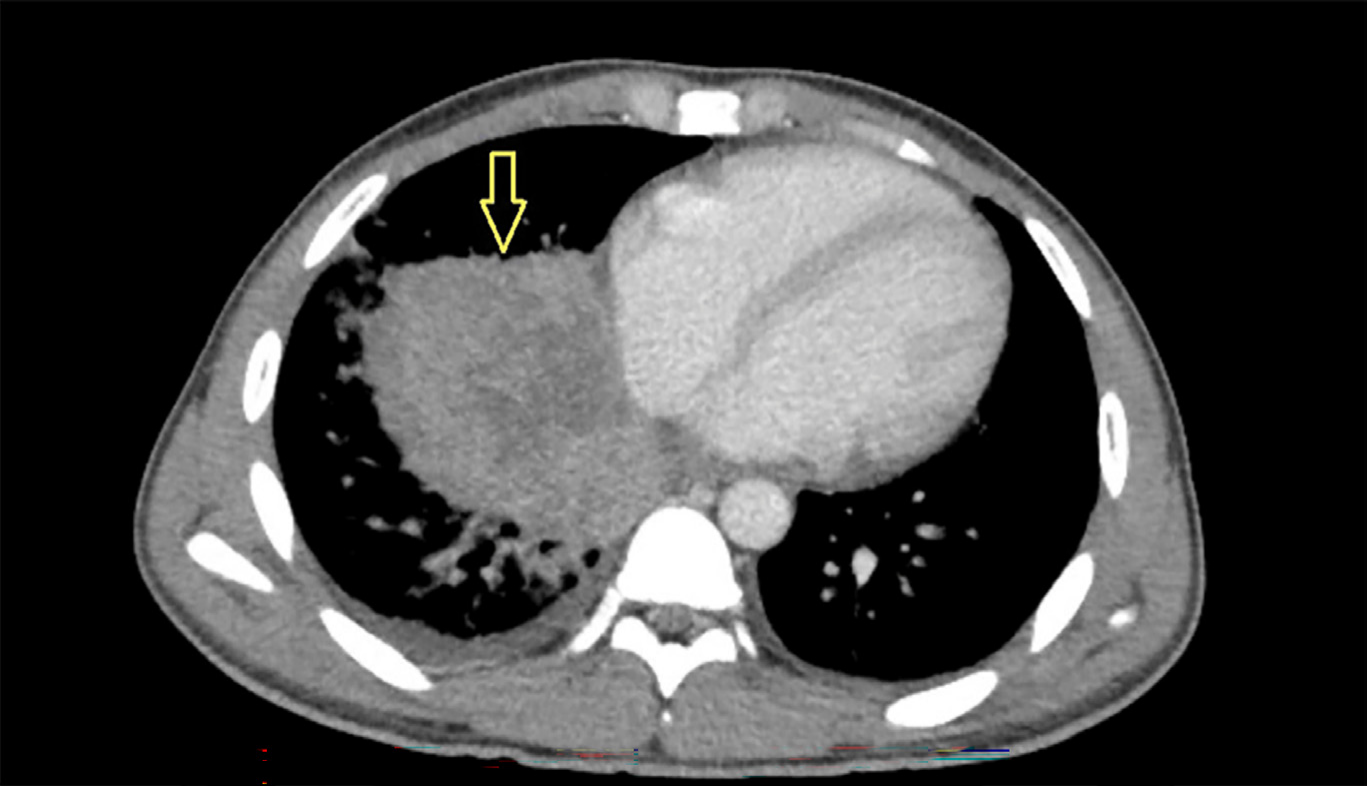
A CT scan with intravenous contrast demonstrating a necrotic lung lesion with small layering right-side pleural effusion.
